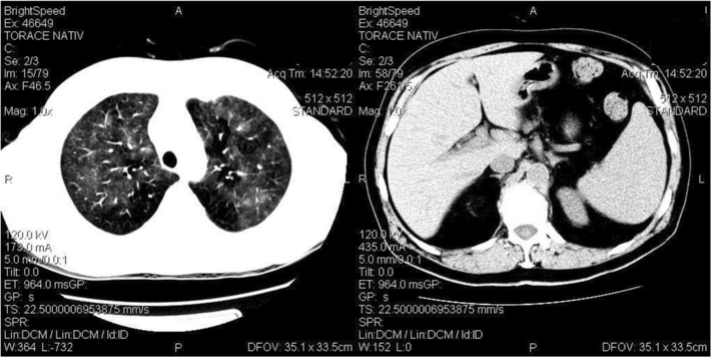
CT scan images. CT images show peripheral and peribronchial patchy ground-glass opacity in both lungs (*left*) and homogenous hepatosplenomegaly (*right*)
